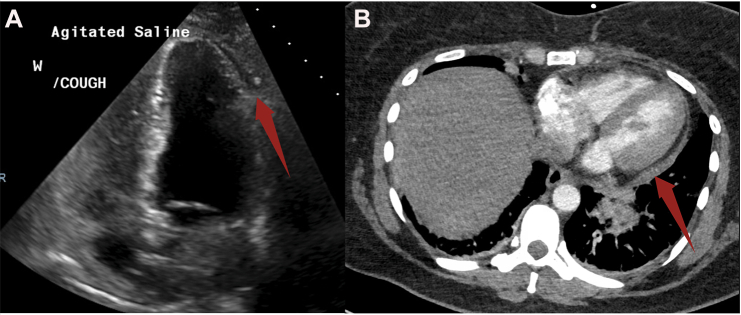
Imaging demonstrating trace pericardial effusion. Postprocedure imaging demonstrating a trace pericardial effusion by transthoracic echocardiogram (TTE) (A) and computed tomography angiography (B).
